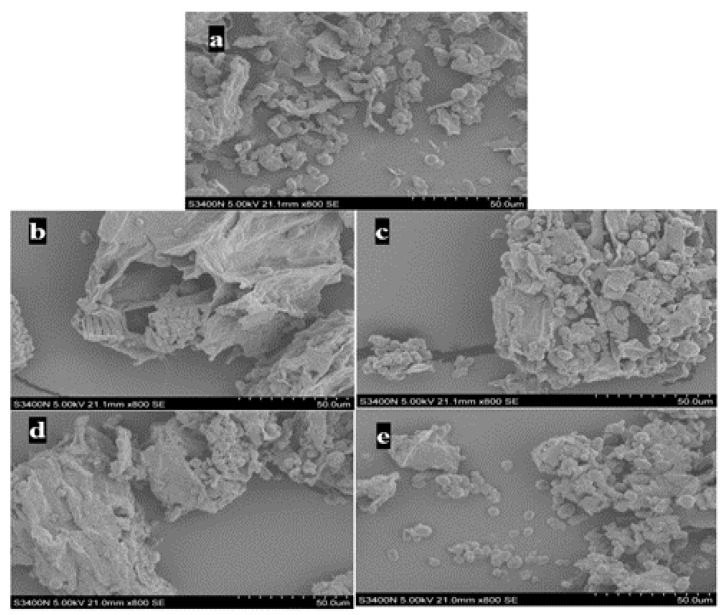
(a) *Glycyrrhiza uralensis* Fisch crude drug powder; (b) *Glycyrrhiza uralensis* Fisch powder obtained by ultrasonic-assisted extraction of DESs; (c) *Glycyrrhiza uralensis* Fisch powder obtained by ultrasonic-assisted extraction of ethanol; (d) *Glycyrrhiza uralensis* Fisch powder obtained by heating and refluxing extraction of DESs; (e) *Glycyrrhiza uralensis* Fisch powder obtained by heating and refluxing the ethanol solution.
